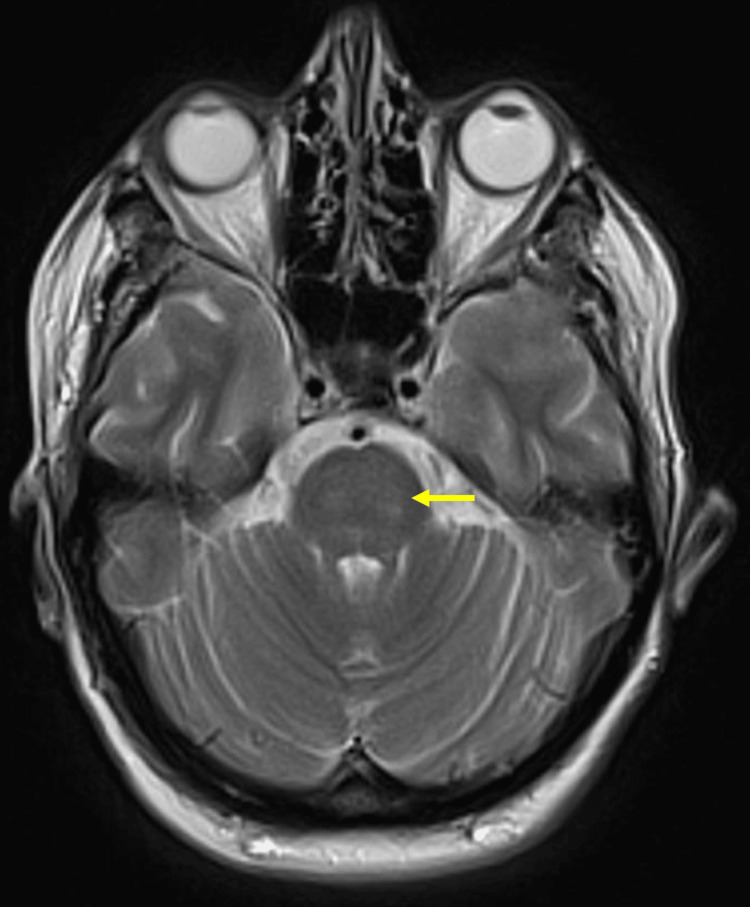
Axial T2-weighted magnetic resonance imaging (MRI) of the brain Axial T2-weighted MRI slice shows a normal, uniform signal within the pons (arrow). There is no evidence of central T2 hyperintensity or the 'Trident sign' characteristic of osmotic demyelination syndrome. This finding effectively ruled out osmotic demyelination as a cause for the patient's prolonged neurological impairment, supporting the diagnosis of severe metabolic encephalopathy secondary to tirzepatide-induced euglycemic ketoacidosis.
